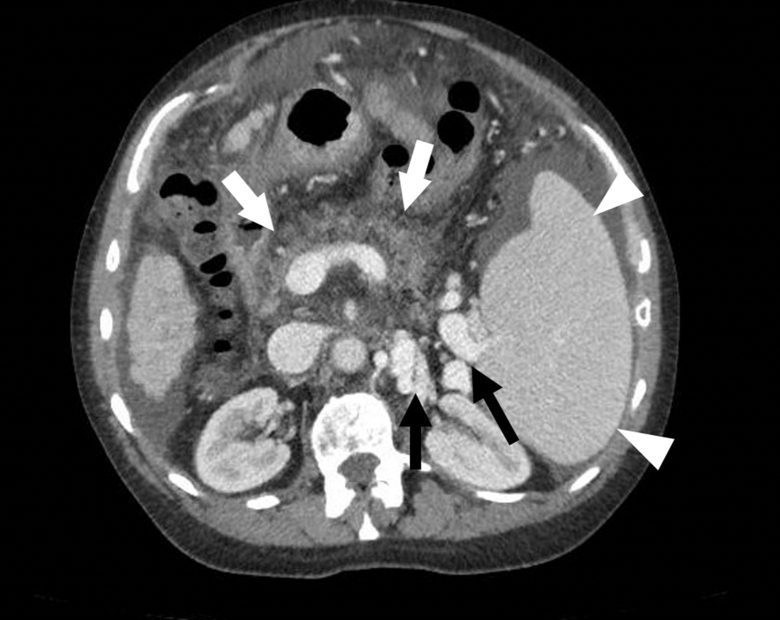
Abdominal CT scan highlights splenomegaly (white triangles), atrophy of the pancreas (white arrows), and varices secondary to portal hypertension (black arrows).
